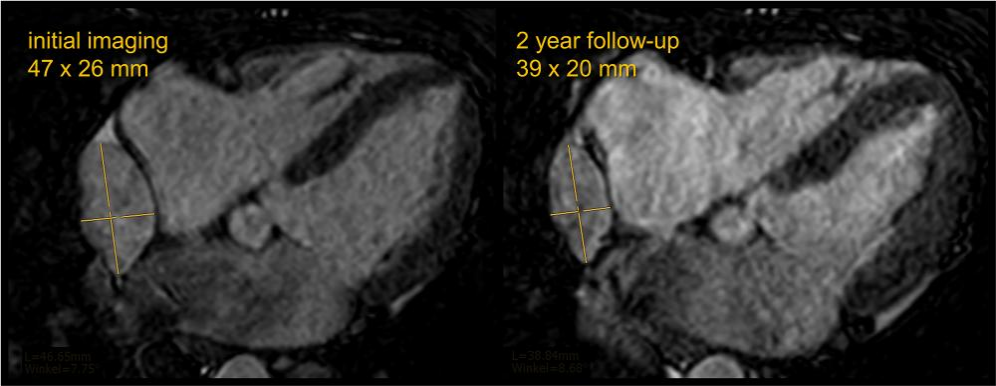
Follow-up by 3D balanced turbo field echo whole-heart imaging at cardiac magnetic resonance imaging, reconstructed in a four-chamber view. 3D balanced turbo field echo images obtained at the initial diagnostic time point (left) and at follow-up 2 years later (right) show a partial regression of this well-circumscribed lesion, which appears isointense to the blood pool.
